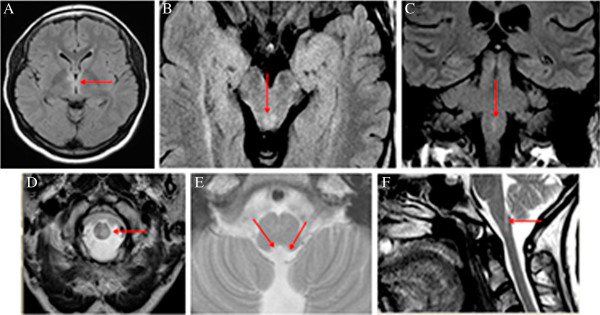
Typical brain MRI lesions in neuromyelitis optica. Representative MRI of six NMO patients; Upper row: FLAIR; lower row: T2W, A. Lesions in hypothalamic region, B. Lesions in periaqueductal matter in mesencephalon, C and D. Lesions in medulla oblongata, E and F. Lesions in area postrema of the medulla oblongata.
